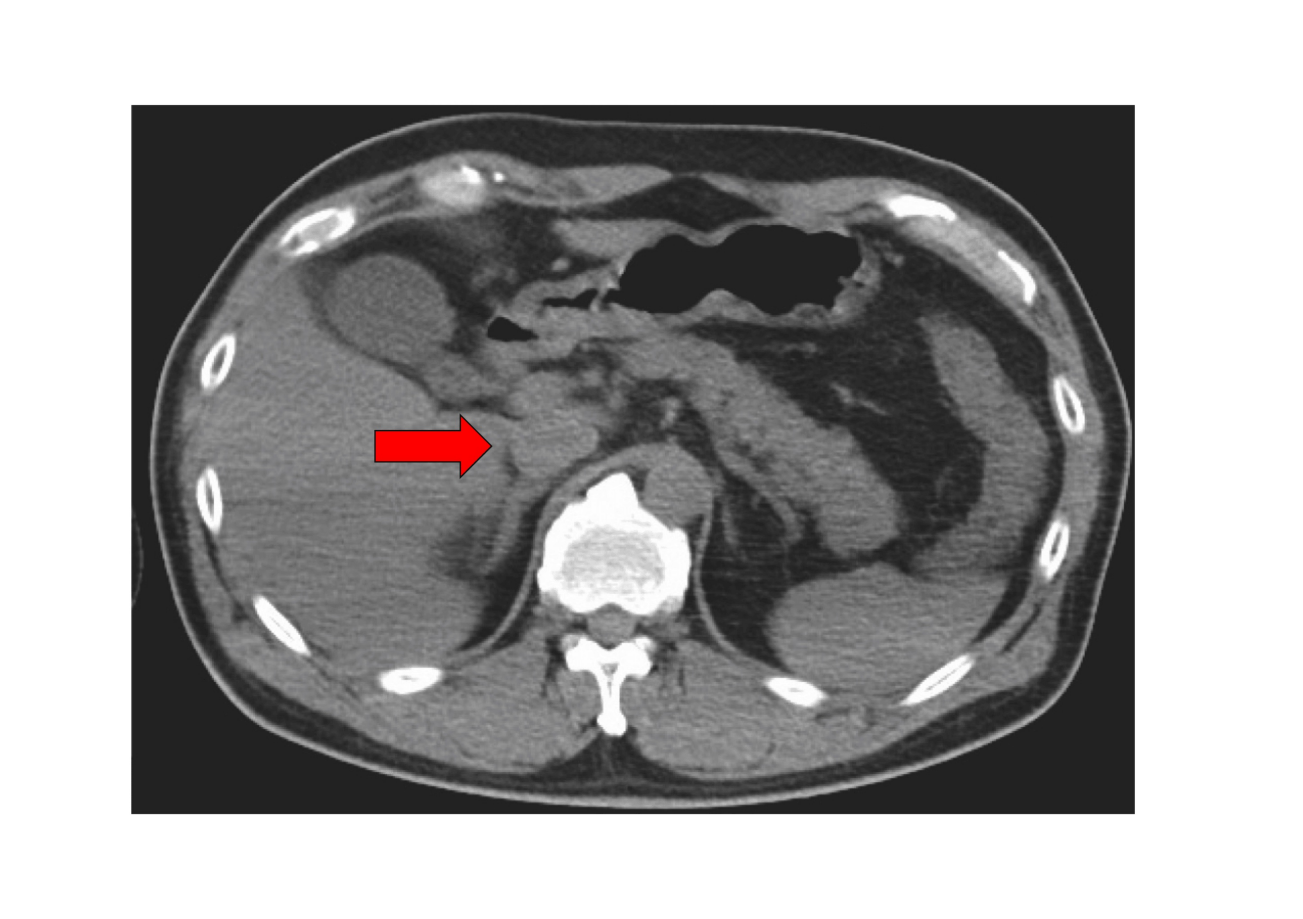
Plain abdominal CT scan A plain abdominal CT scan performed upon the patient's arrival at the hospital showed that the inferior vena cava (red arrow) was neither distended nor collapsed, with no obvious abnormalities detected. These findings suggest that the patient was not significantly dehydrated despite exposure to high temperatures.
